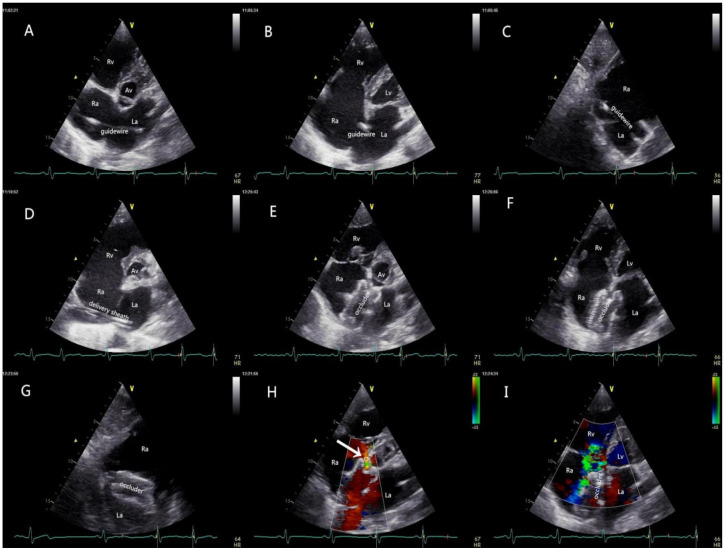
Procedural protocol. (A) The guidewire was inserted from the RA into the LA via the femoral vein under the guidance of TTE; (B,C) the tail end of the high-echo guidewire was confirmed to be inside the LA in the four-chamber view and the short-axis subcostal view; (D) a delivery sheath was inserted along the guide-wire into the LA, and then the inner core and the guidewire were withdrawn; (E) the occluder was delivered carefully through the sheath under the real-time guidance of TTE, and the LA disc was opened; (F,G) the occluder was completely released, and the two discs of the occluder were accurately parallel to the plane of the atrium septal; (H) the blood flow from CS was fluent (white arrow); (I) the shunt between RA and LA disappeared, and no mitral regurgitation was detected with TTE. Mild tricuspid regurgitation still existed, as confirmed before the interventional catheterization. TTE: transthoracic echocardiography; RA: right atrium; LA: left atrium; RV: right ventricle; LV: left ventricle; TV: tricuspid valve; AV: aortic valve; CS: coronary sinus.
